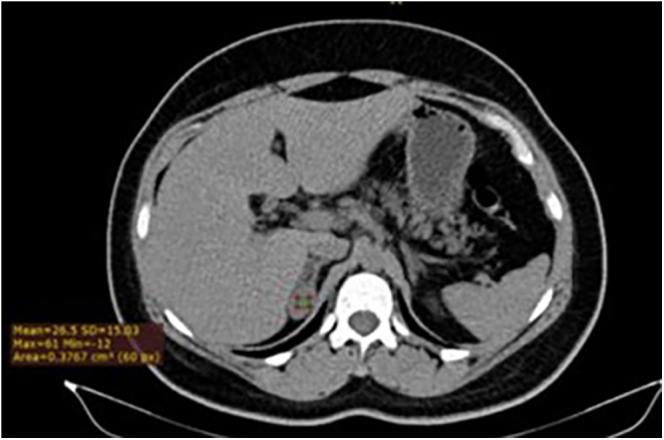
Abdominal CT scan without contrast demonstrates a nodular image in the right adrenal gland, measuring 1.9 × 3.1 cm and 26 HU.
